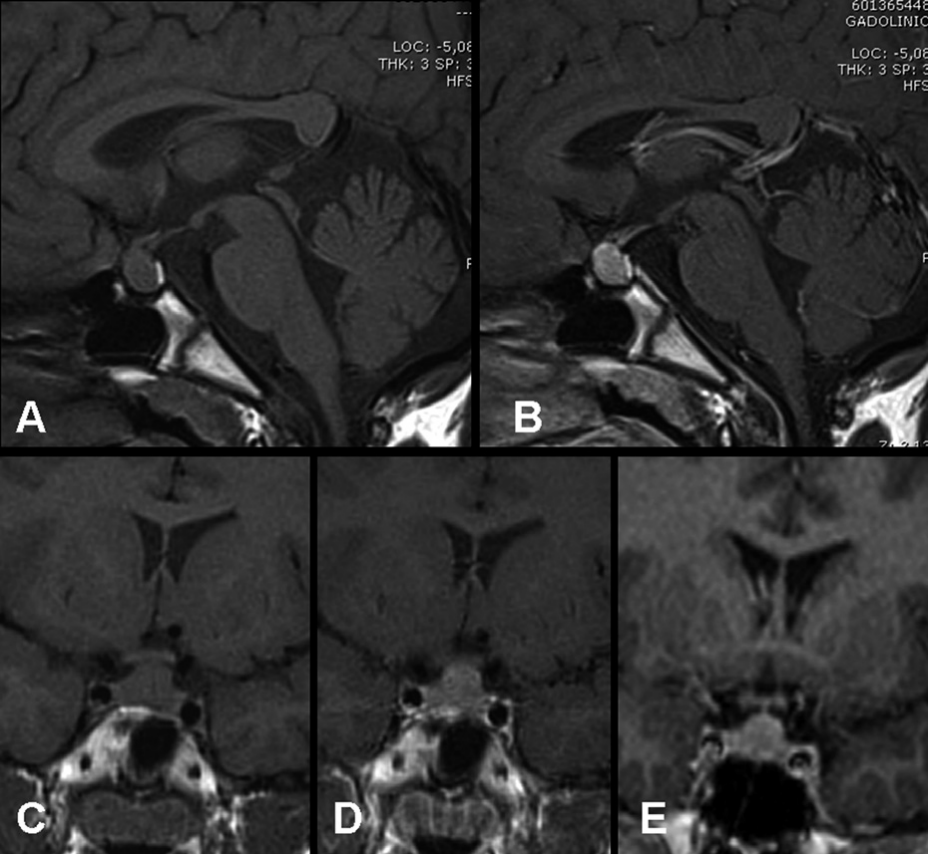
A-E. Pretreatment sagittal (A, B) and coronal (C-E) T1-weighted MR images show diffuse enlagement of the pituitary gland, that extends into the suprasellar cystern with mild compression of the optic chiasm and of the posterior neurohypophyseal lobe, which keeps its normal bright signal. After intravenous Gadolinum administration (B, D) the mass demonstrate homogeneous enhancement; the pituitary stalk (E) is raised but still along the midline.
